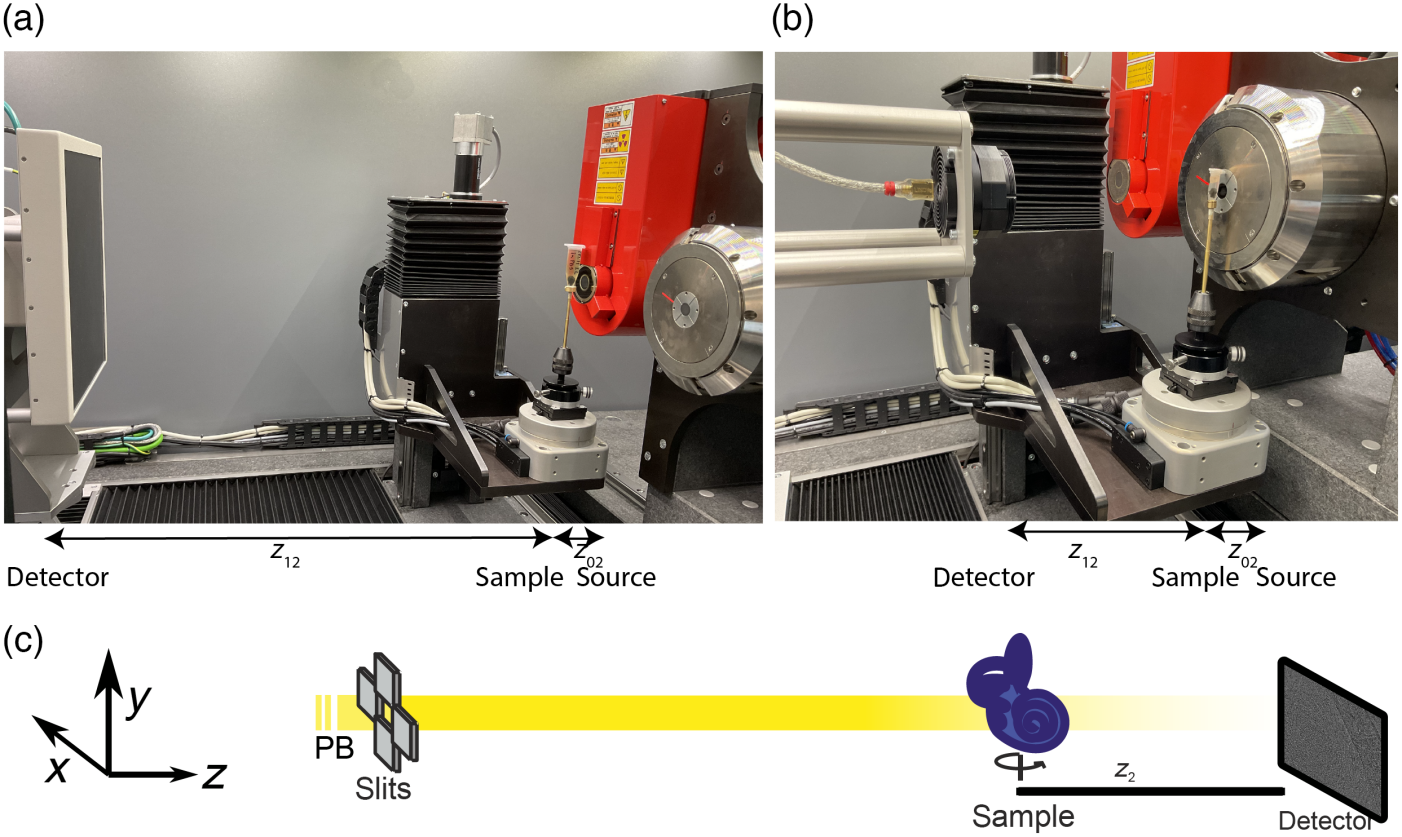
Experimental setups. Laboratory μ-CT instrument (EasyTOM, RX Solutions) with (a) microfocus source and flat panel detector and (b) nanofocus source and CCD detector. The system can be operated in all source–detector combinations. (c) Synchrotron parallel beam setup at the GINIX endstation of the P10 coherence beamline (PETRA III, DESY).
